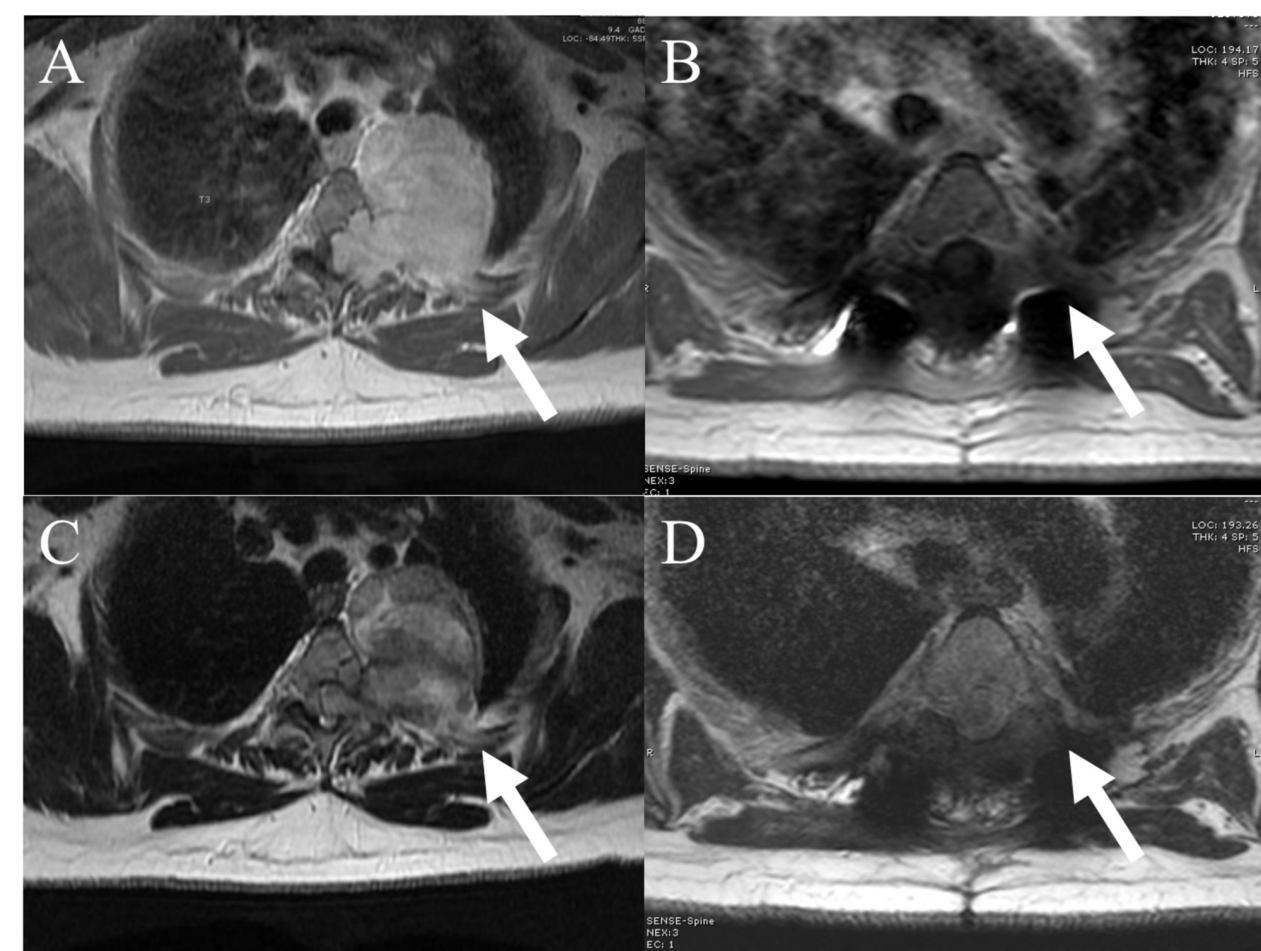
Comparison of preoperative and postoperative MRI thoracic spine with and without contrast Comparison of pre-operative T1 with gadolinium axial MRI (A) with post-operative TI with gadolinium axial MRI (B). Comparison of pre-operative T2 axial MRI (C) with post-operative T2 axial MRI (D). MRI, magnetic resonance imaging
